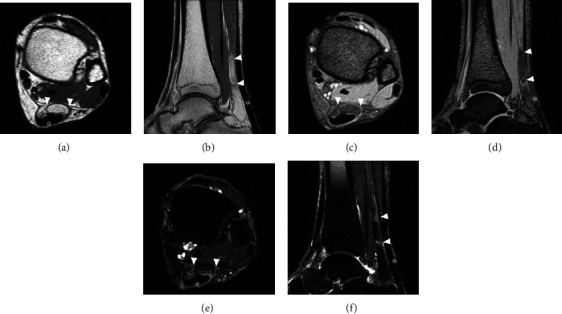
Magnetic resonance (MR) imaging at the second visit. Axial (a, c, and e) and sagittal (b, d, and f) images of T1WI, T2WI, and T2FS MR images. The second MR image shows that the lesion has migrated to the ventral aspect of the Achilles tendon. The location and shape of the lesion has changed compared with the previous findings on the previous MRI, suggesting that the lesion was moving in and out around the Achilles tendon.
